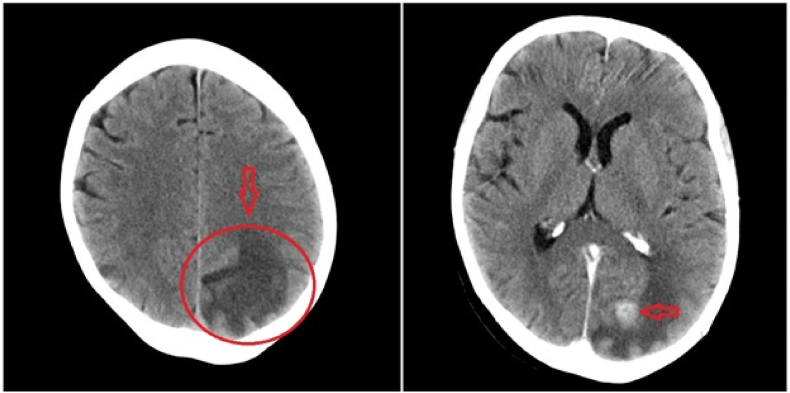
A brain CT scan, non-contrast multiple areas of decreased attenuation in the bilateral occipital lobes and the right parietal lobe (red arrows).
